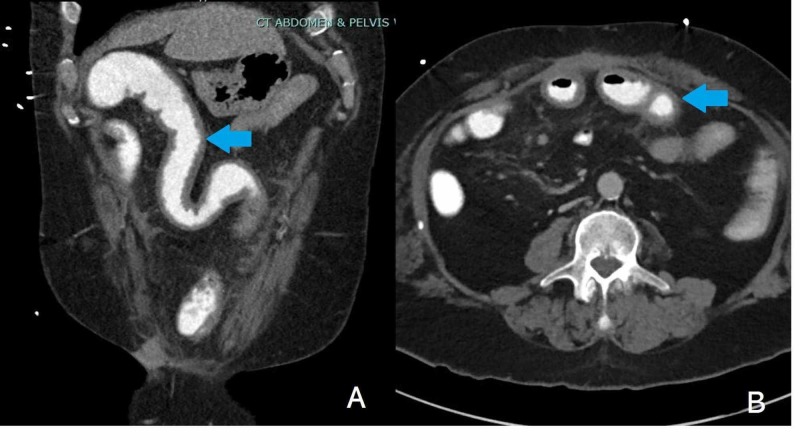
CT abdomen and pelvis with oral contrast showing bowel thickening with minimal stranding, suggestive of enteritis in coronal cut (blue arrow, A) and axial cut (blue arrow, B) CT: computed tomography
